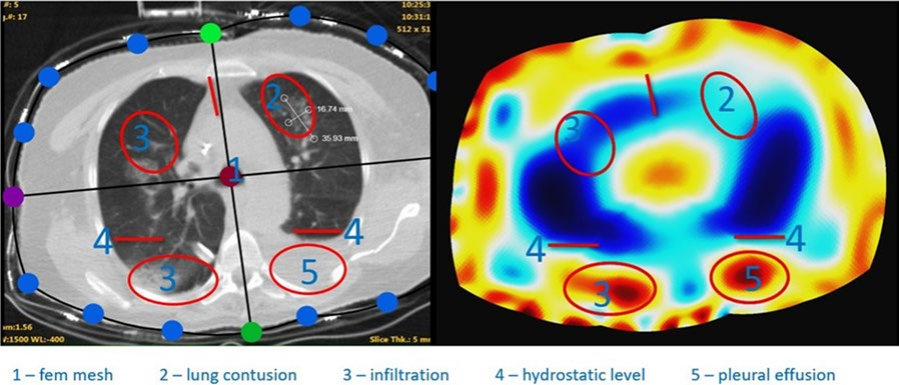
Comparison between CT scan and the resultant EIT reconstructed image.
